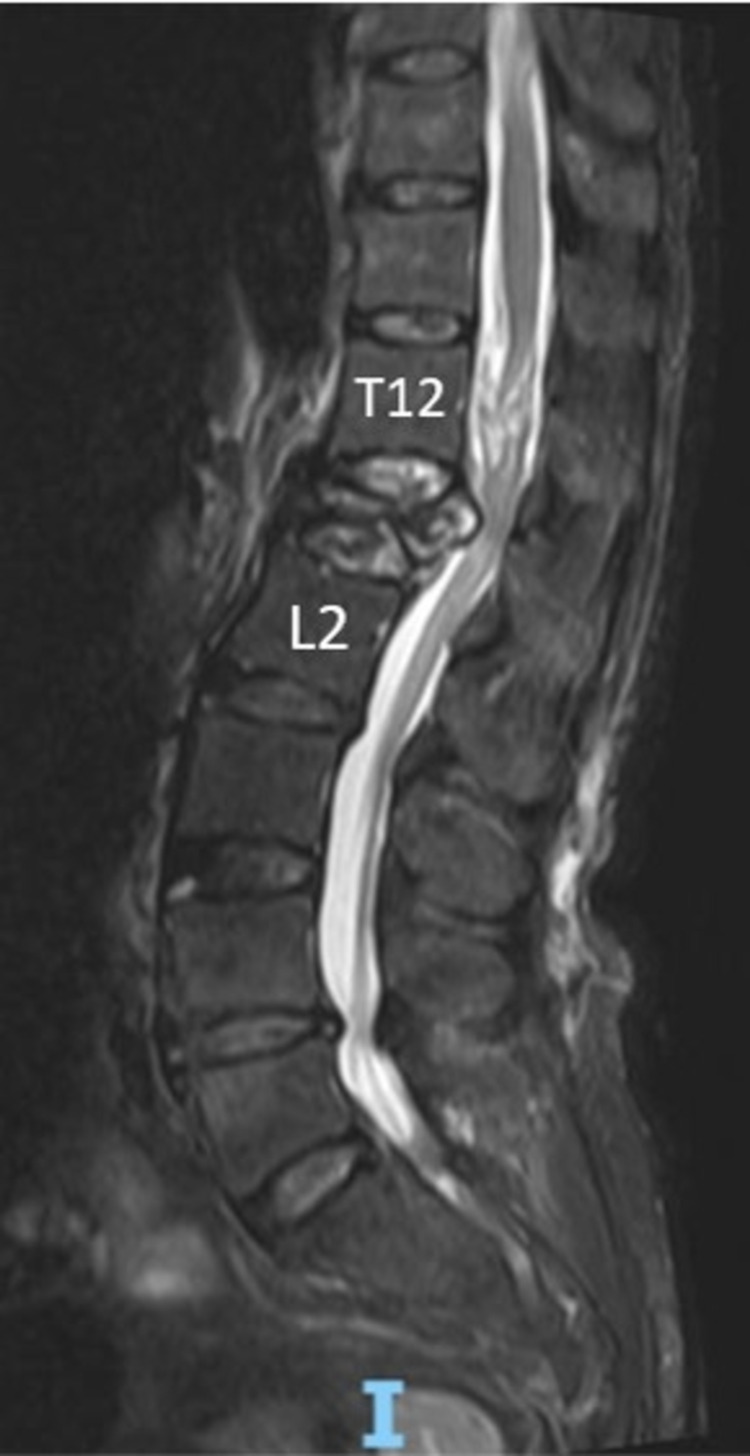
Preoperative T2-weighted MRI of the lumbar spine - sagittal view
